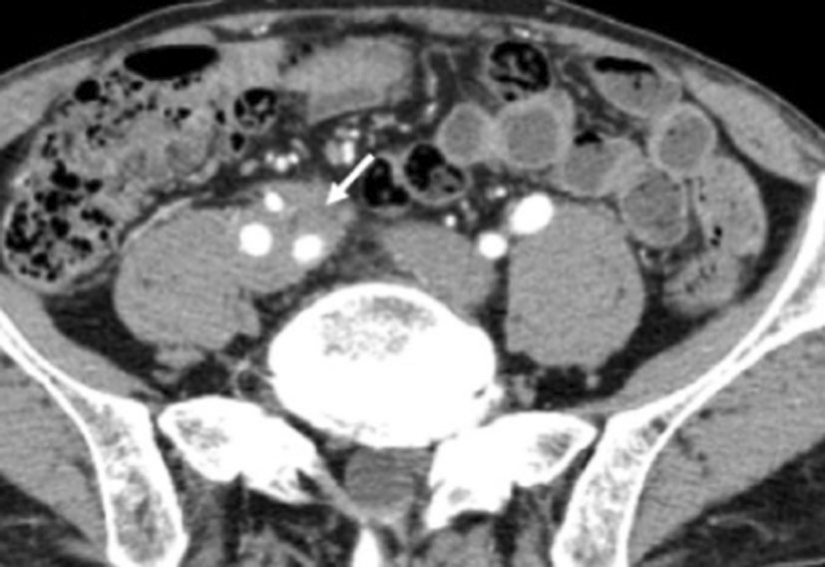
Contrast-enhanced CT images of IgG4-related periaortitis/periarteritis. Contrast-enhanced CT (aortic phase) shows soft-tissue mass (arrow) around the right iliac artery
